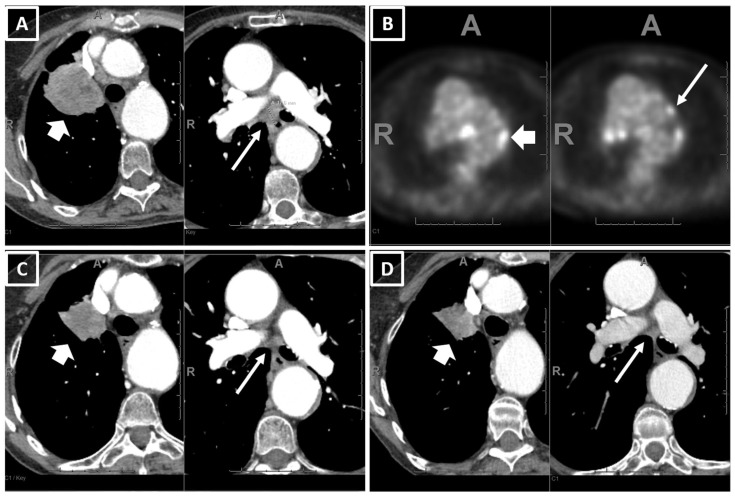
An example of a patient who had PR with pembrolizumab monotherapy and had a low value for the predicted risk of progression (0.03) using the prediction equation and the Youden cut-off. A 79-year-old female, ex-smoker, with 45 pack years, ECOG 1, diagnosed as stage IIIC NSCLC (adeno.). (A) The baseline CT showed a 6.0 (T3) right upper lobe primary lung tumor and 1.8 cm short axis subcarinal adenopathy (T3N2, short and long arrows in (A)), while (B) PETCT showed contralateral hilar and mediastinal adenopathy suggestive of N3 (short and long arrows in (B)). (C) The 1st FU CT after 11.5 weeks on pembrolizumab showed a partial response (PR) (RUL tumor decreased to 3.2 cm and LN decreased to a 0.7 cm short axis), and (D) the 2nd FU CT after 28 weeks showed a stable disease. The patient showed early signs of disease progression on the CT after 54 weeks on pembrolizumab.
